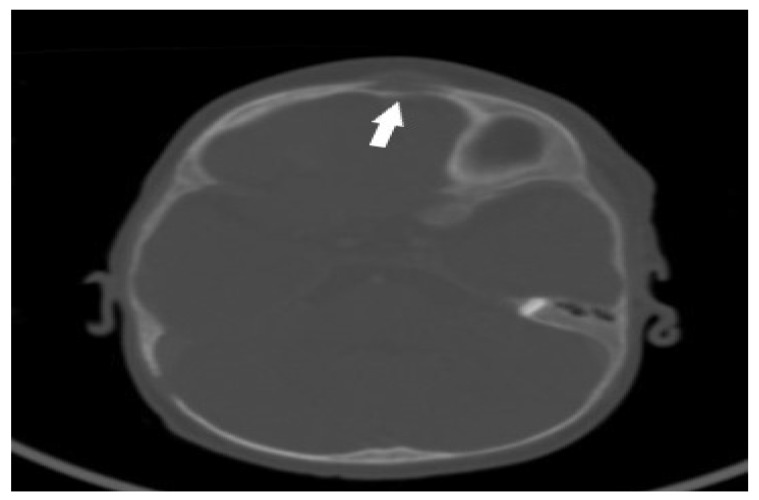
CT image of the head—frontal osteolytic bone lesion with soft tissue infiltration (white arrow).
